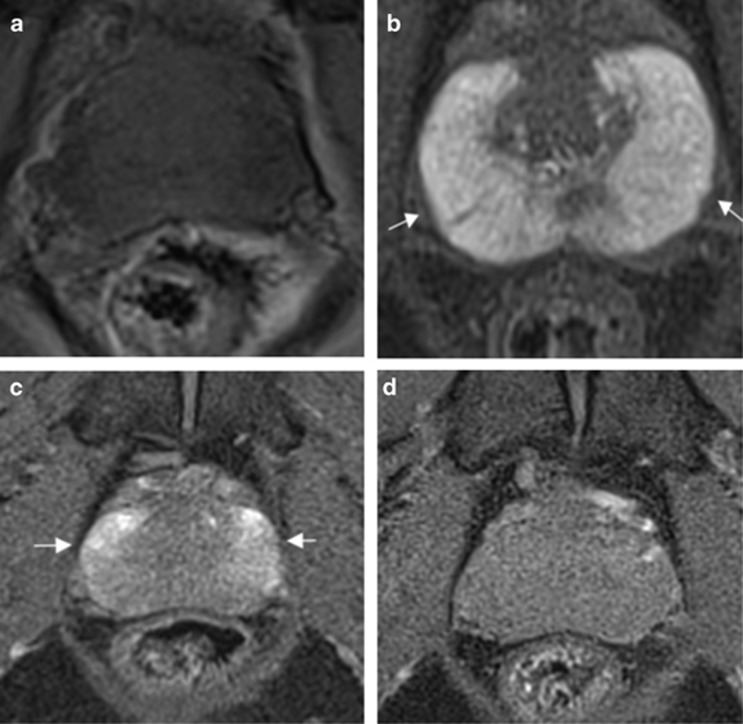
Representative axial T1-weighted images at the mid-gland level demonstrate (a) normal peripheral zone T1 signal intensity pre-biopsy; and increase (white arrows) in the peripheral zone T1 signal intensity at (b) 1 month, (c) 2 months and (d) 6 months post biopsy.
